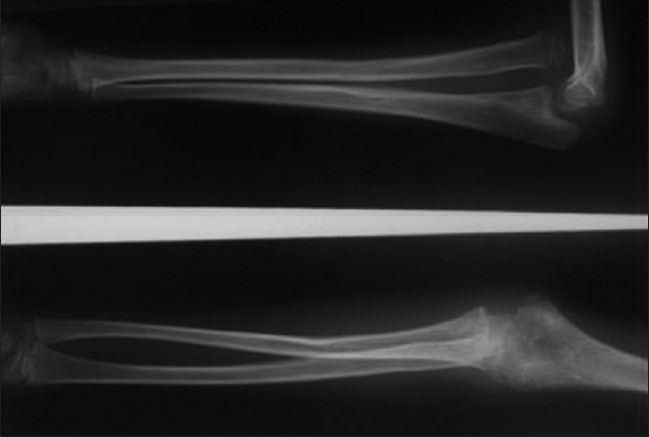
Lateral and anteroposterior radiograph of right elbow of a seven year old girl showing missed Monteggia fracture of 18 months
